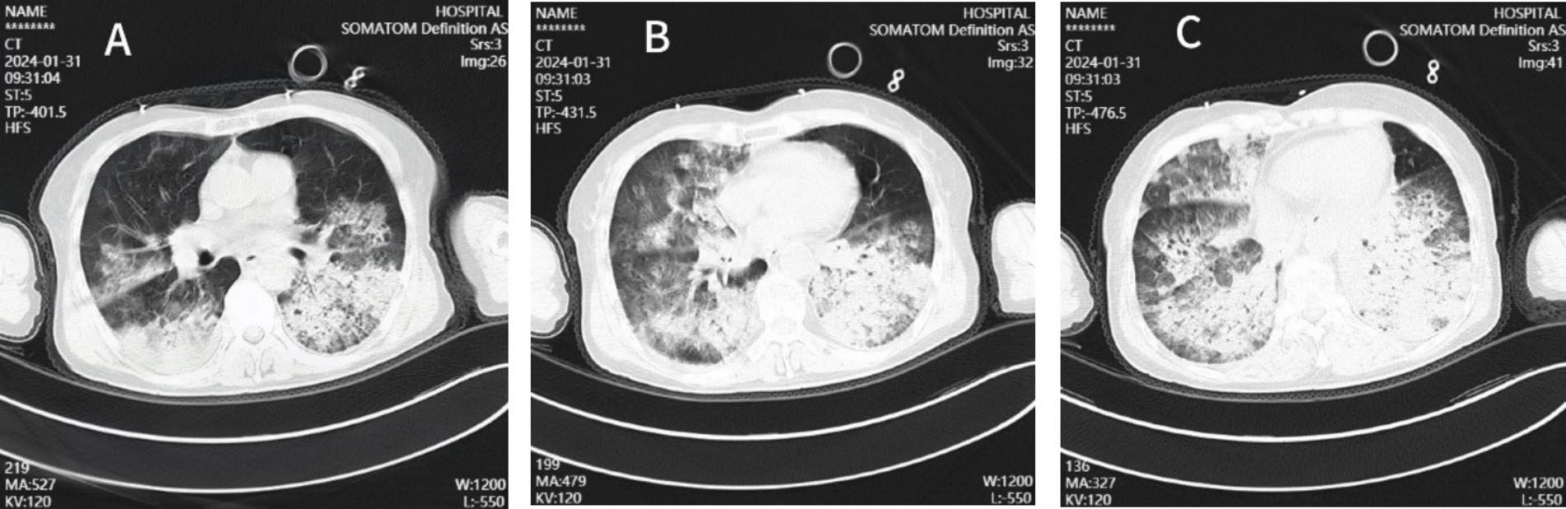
Chest CT images of the patient. The CT chest images (A–C) showed patchy shadows and ground-glass shadows in the lungs.
